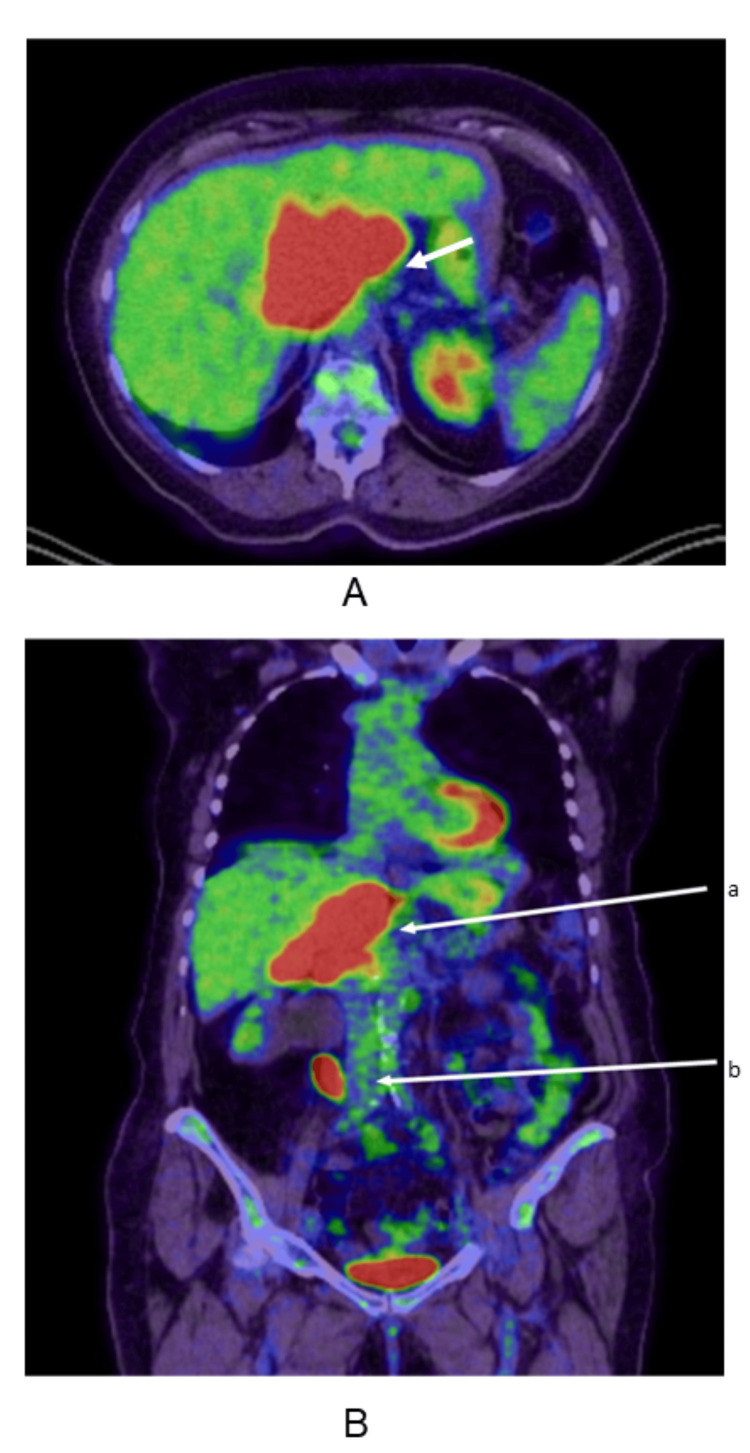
PET-CT scan (A) Axial PET-CT image showing increased FDG avidity in the periportal region(arrow). (B) Coronal PET-CT image depicting (a) FDG-avid periportal lymph nodes and (b) right ureteric soft tissue thickening PET-CT: positron emission tomography-computed tomography; FDG: fluorodeoxyglucose
